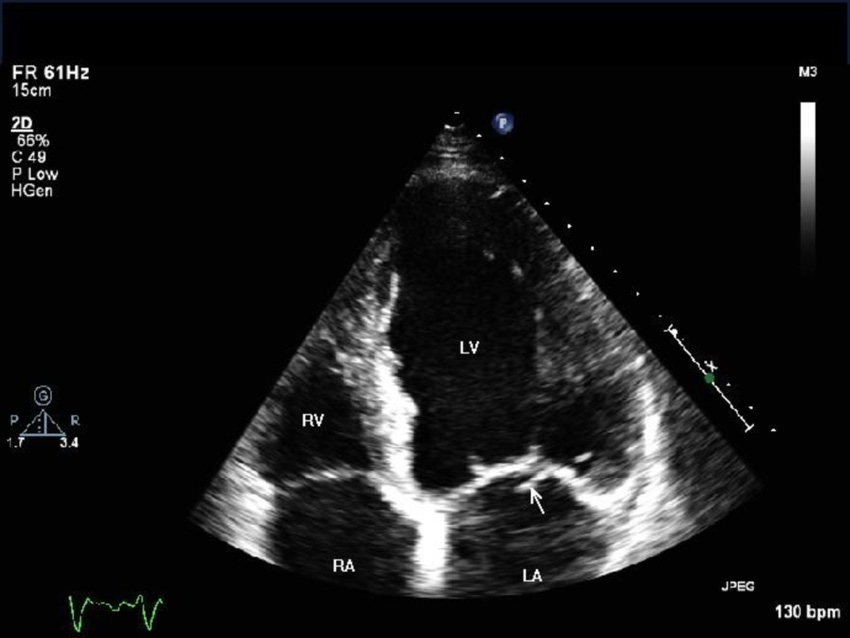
Echocardiogram (apical view) showing vegetation in the native posterior mitral valve leaflet (white arrow). LA = left atrium; LV = left ventricle; RA = right atrium; RV = right ventricle.
